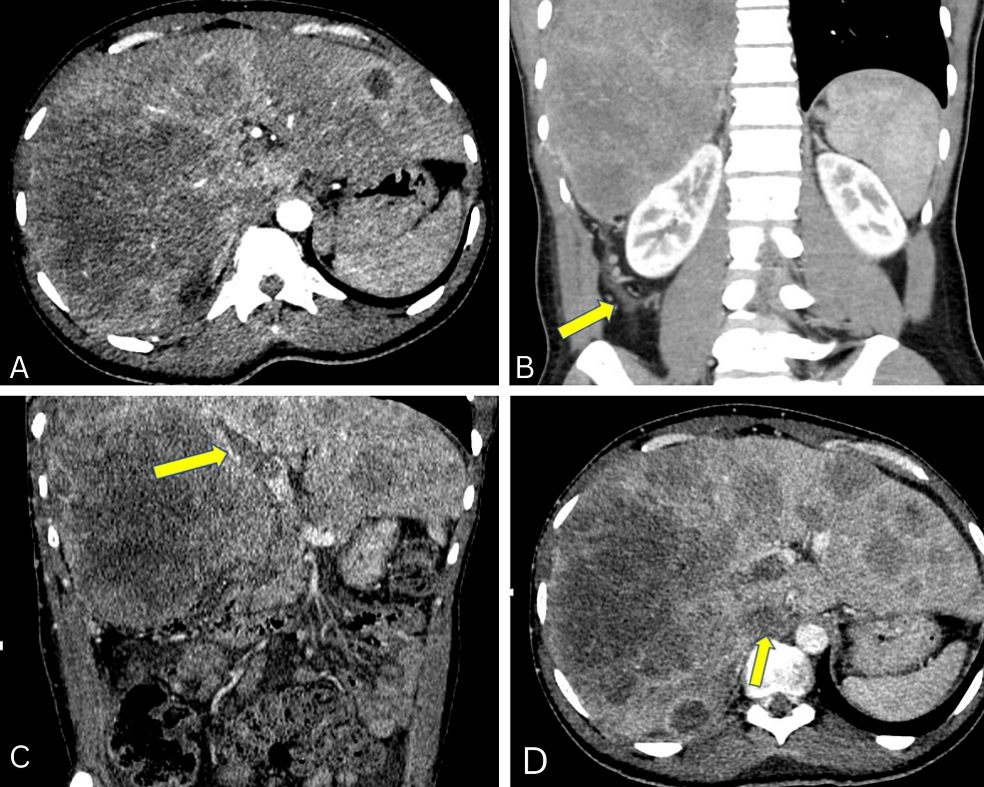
CT abdomen of the patient. (A) Axial section shows innumerable hepatic masses replacing the entire right hepatic lobe, involvement of greater than 50% of the left hepatic lobe, (B) coronal section shows omental metastasis (arrow), (C) coronal section shows portal vein tumor thrombus (arrow), and (D) axial section of CT scan showing intrahepatic IVC involvement (arrow). CT: computed tomography; IVC: inferior vena cava
